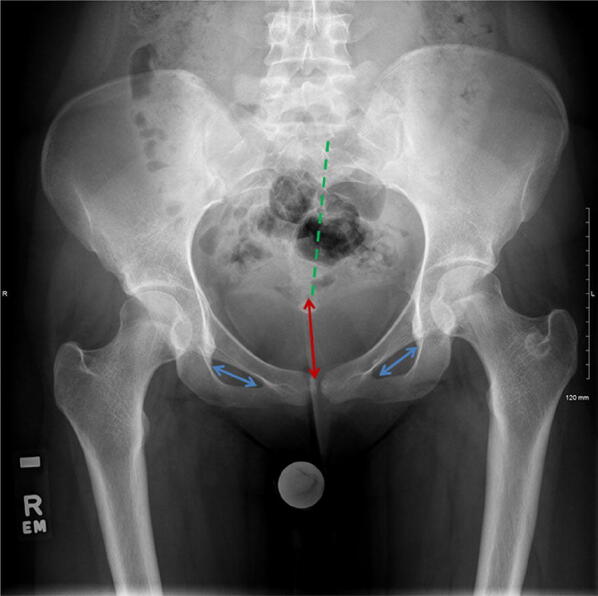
AP radiograph pelvis depicting pelvic rotation and tilt. The central sacral line (green dotted line) should be aligned centrally from the tip of the coccyx to the symphysis pubis. The obturator foramina (blue arrows), ischial spine and trochanters should be symmetrical. In this case, there is a slight pelvic rotation. The distance between sacro-coccygeal junction and the superior end of the symphysis (red arrow) is used to determine the pelvic tilt. A benign sclerotic ring is seen in the left trochanteric region
